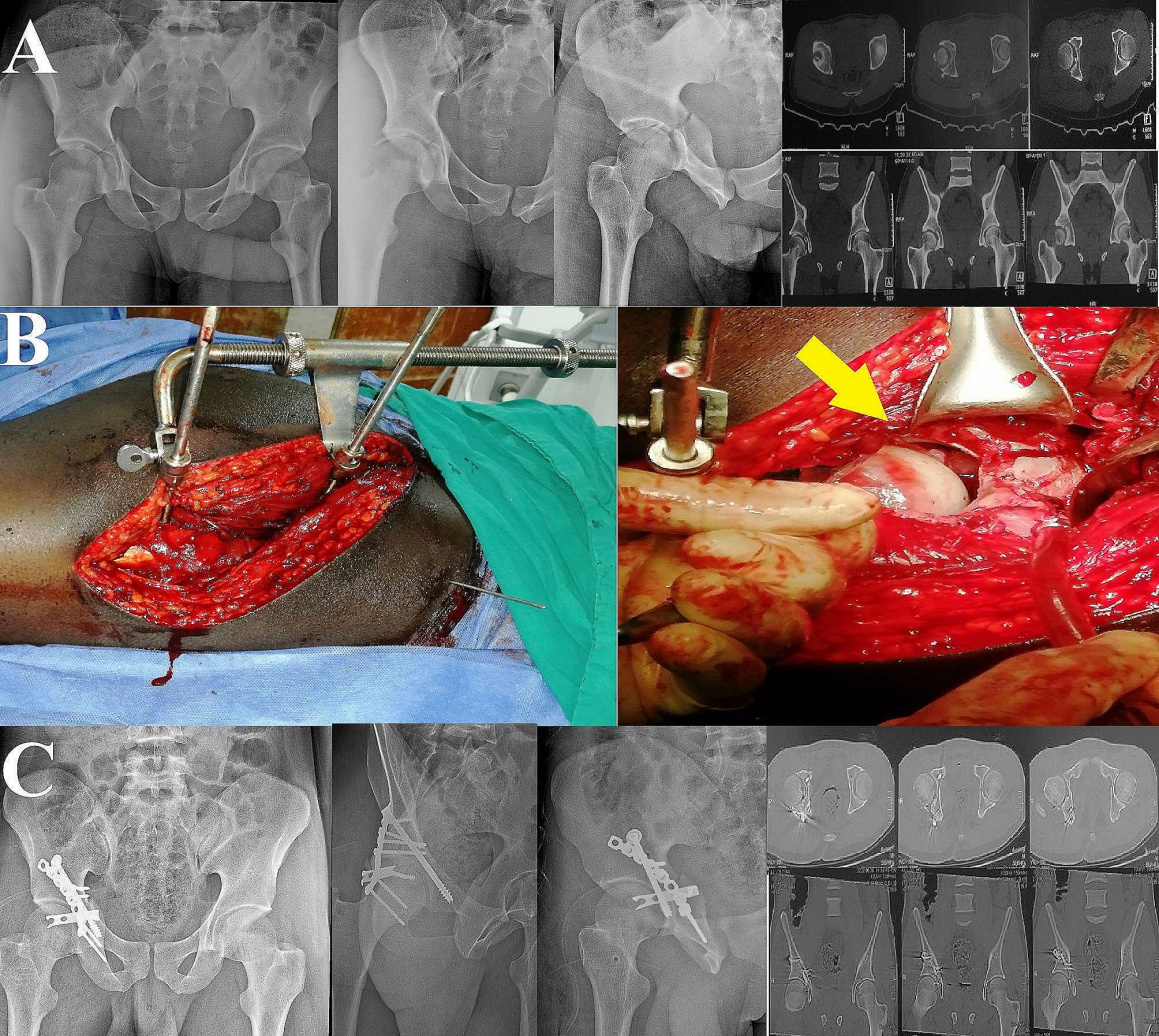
A male patient, 26 years old, presented with a Transverse fracture of the right acetabulum associated with a posterior wall fracture associated with an incarcerated intraarticular fragment. A, preoperative plain radiograph and CT scans. B shows the intraoperative application of the femoral distractor and the amount of hip joint distraction (yellow arrow). C, post-operative plain radiographs and CT scans showing fracture anatomical reduction and clearance of the hip joint space
